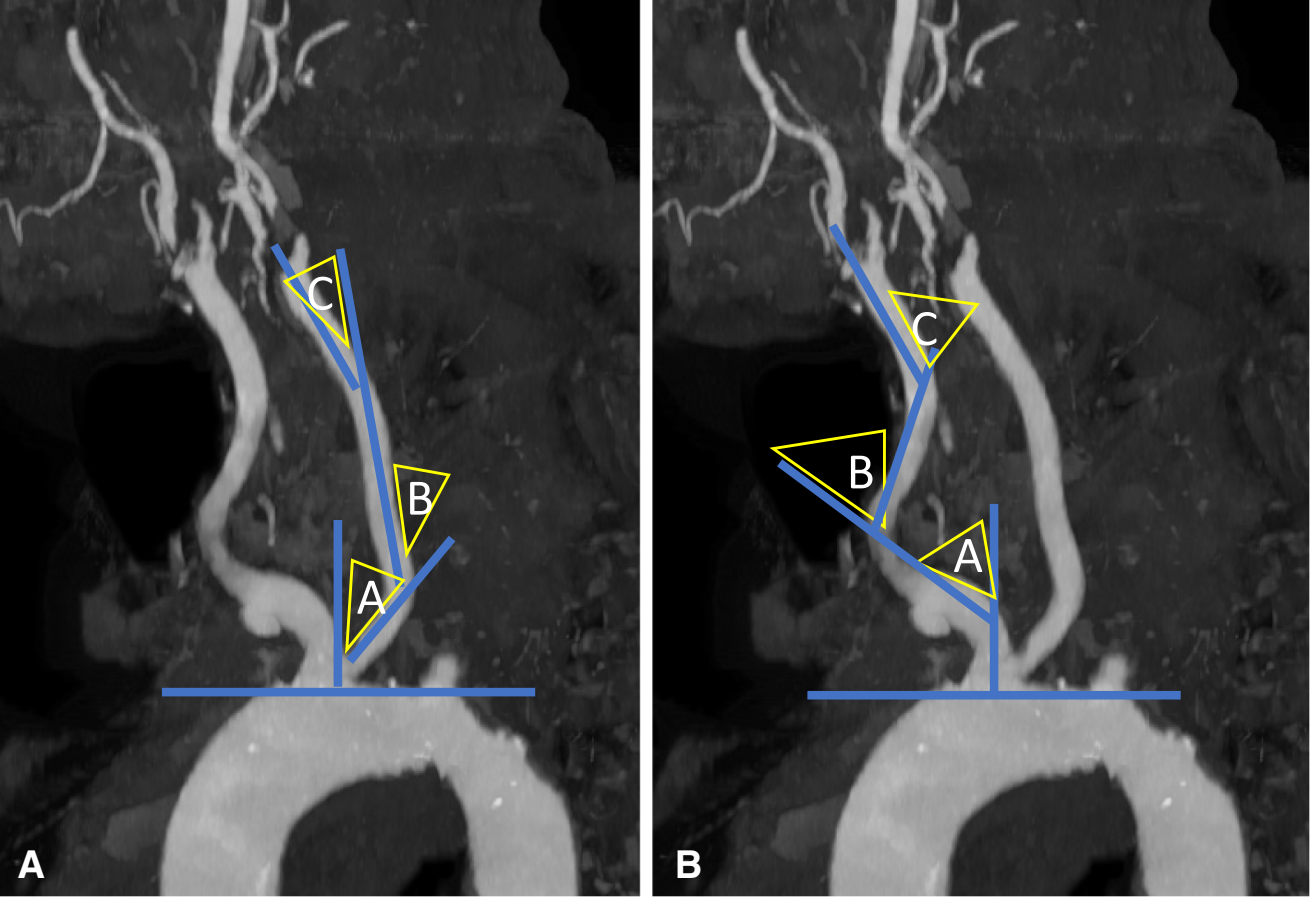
CT angiography with maximum intensity projection in left anterior oblique view. Proximal tortuosity index (TI), A right and B left, is the mean of A + B + C. C Intra-arterial digital subtraction angiography of the right carotid artery. Distal tortuosity index (TI) is the sum of A + B + C angles
